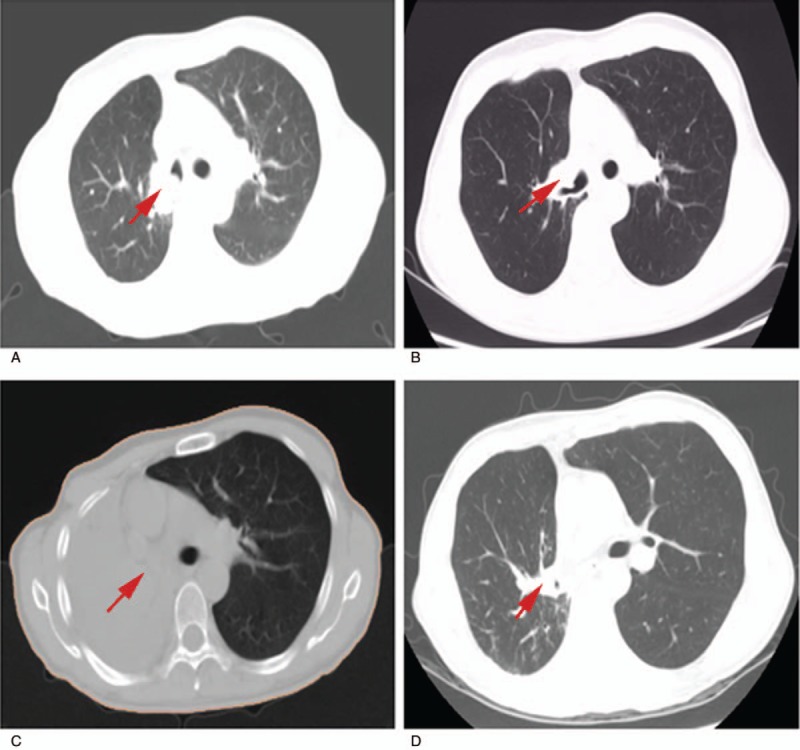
Chest computed tomography of the lung tumor patient undergoing repeat radiotherapy. (A) Recurrence of disease in the hilum of the right lung after surgery. (B) Partial response at the end of the first radiotherapy. (C) Recurrent disease again in the right lung. (D) After repeat radiotherapy, the enlarged tumor in the right lung shrank significantly and the atelectasis nearly disappeared.
